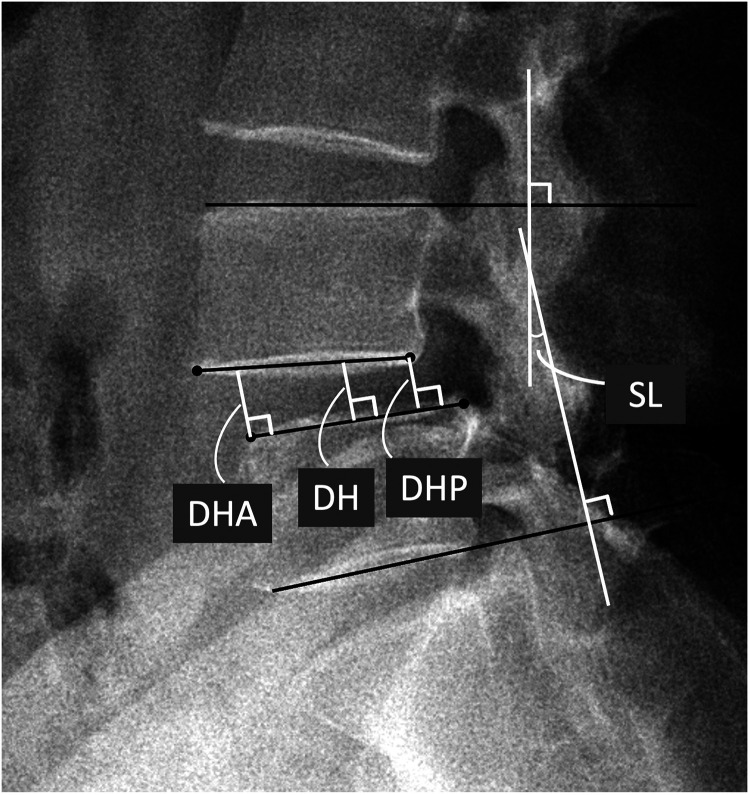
Definitions of radiographic parameters. SL, DH, DHA, and DHP on the standing lateral view of lumbar spine. SL, segmental lordosis; DH. disc height; DHA, anterior DH; DHP, posterior DH.
